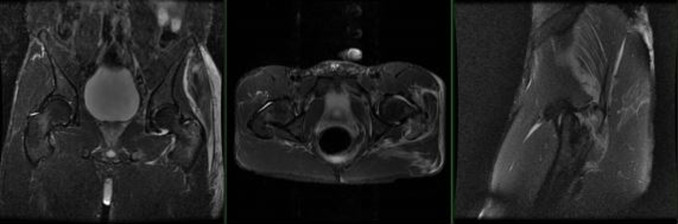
1.5 Tesla MRI of the pelvis demonstrating (coronal, axial, and sagittal slices) an isolated greater trochanteric left hip fracture with minimal displacement and no intertrochanteric extension. Associated left gluteus medius tendinosis without avulsion of the tendinous insertion on the greater trochanter fragment. Increase fluid intensity throughout the gluteus medius and maximus muscle bellies.
